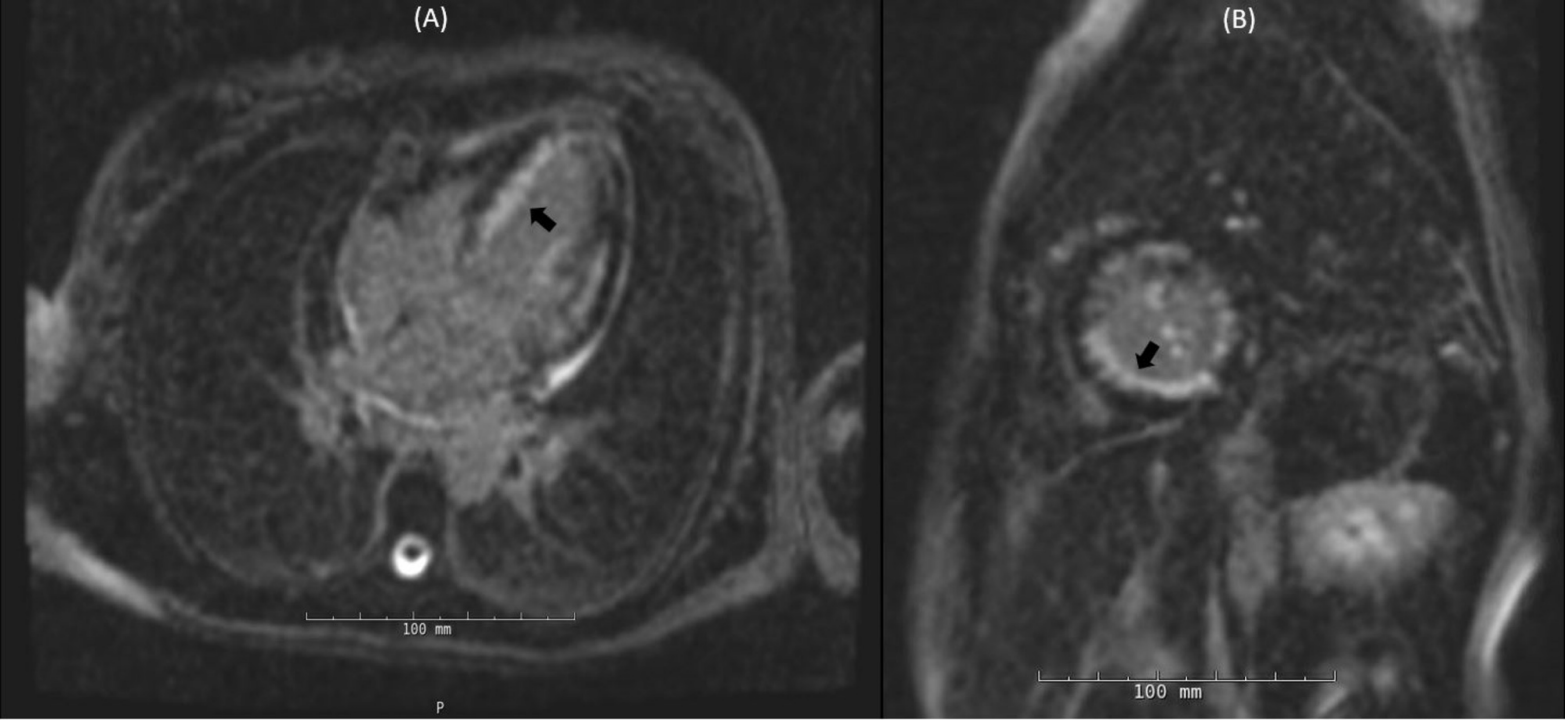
Cardiac magnetic resonance imaging during current hospitalization. Diffuse late gadolinium enhancement (black arrow) in four chamber view (A) and short axis view (B).
